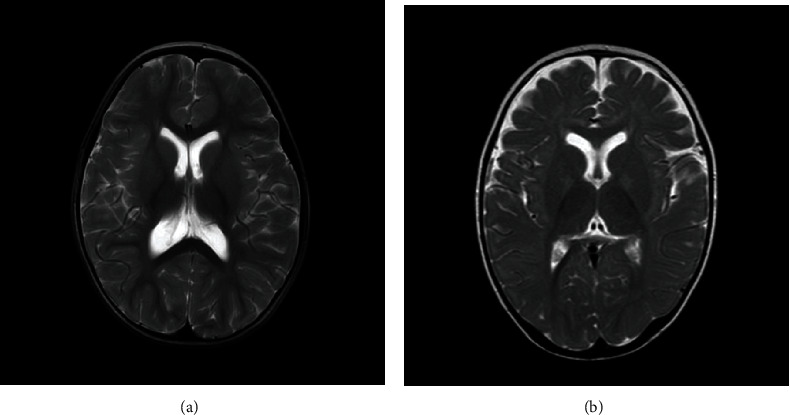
(a) Cranial MRI of Patient 1 showed hypomyelination and enlargement of lateral ventricles. (b) Cranial MRI of Patient 2 showed partial T2W signal in bilateral pallidus that was slightly higher, and some extracerebral fluid spaces were wider.
